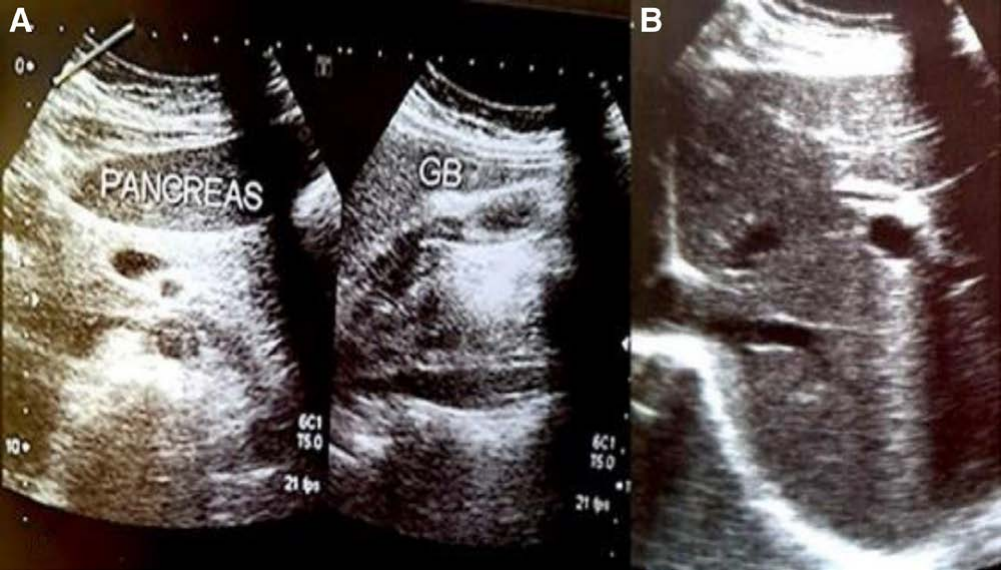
Abdominal ultrasound for a 47-year-old woman showed a normal pancreas, gallbladder (A) and liver (B).
